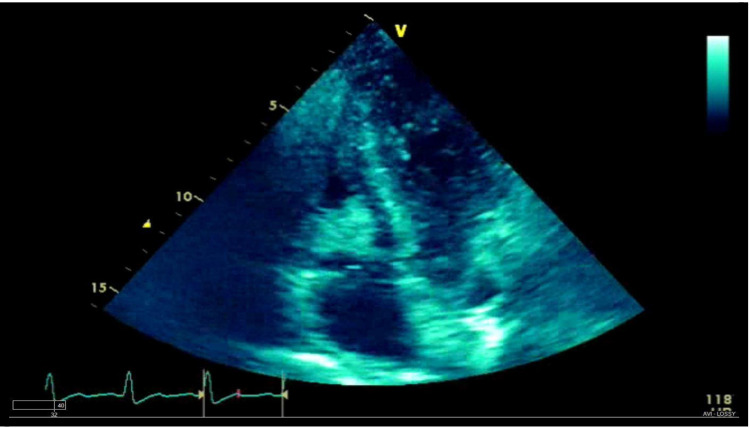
Transesophageal echocardiogram with Definity® contrast perfusion study showing contrast uptake by the right ventricle (RV) mass The mass in the right ventricle (RV) has irregular borders and shows significant contrast uptake.
